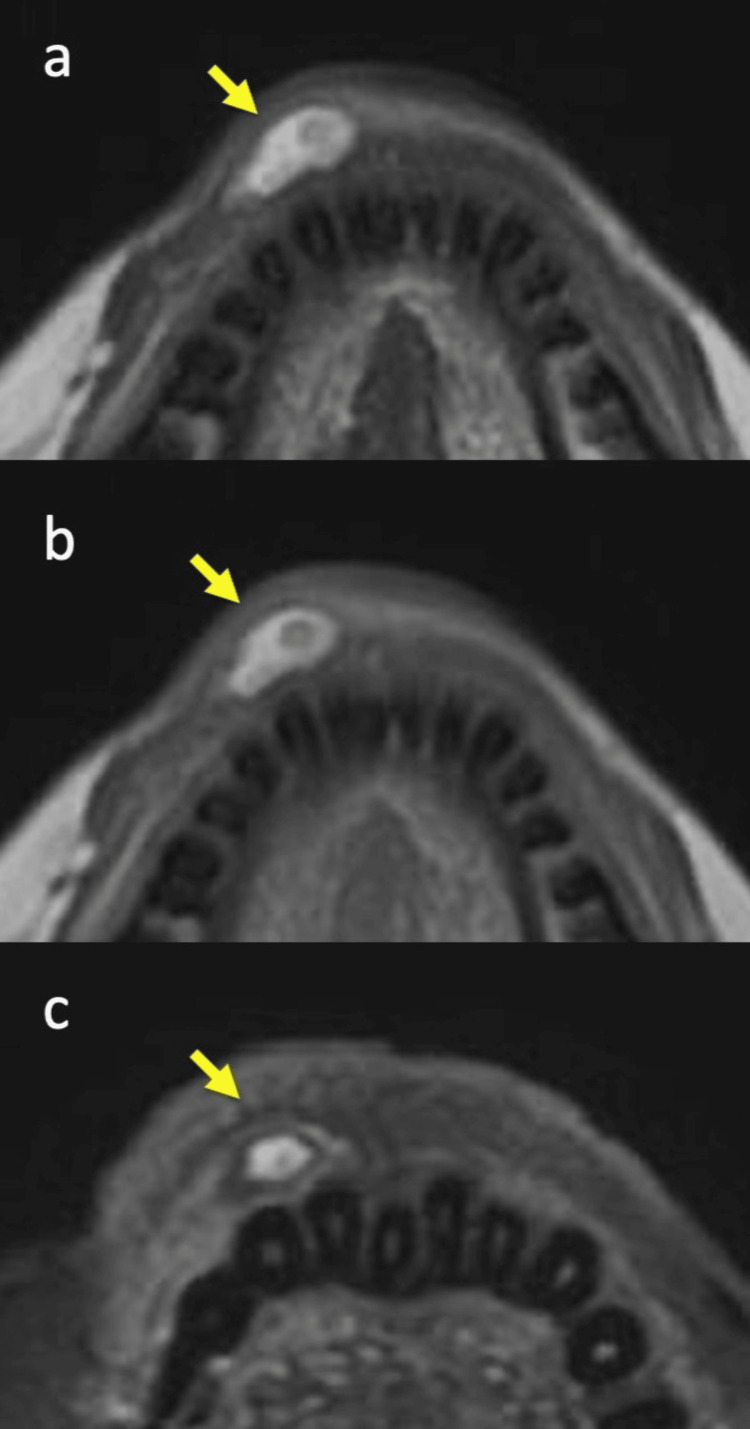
Magnetic resonance imaging (MRI) of the lower lip showing the tumor’s position (indicated by arrows). (a) T1-weighted axial image; (b) T2-weighted axial image; (C) Fat-suppressed axial image by short tau inversion recovery (STIR).
